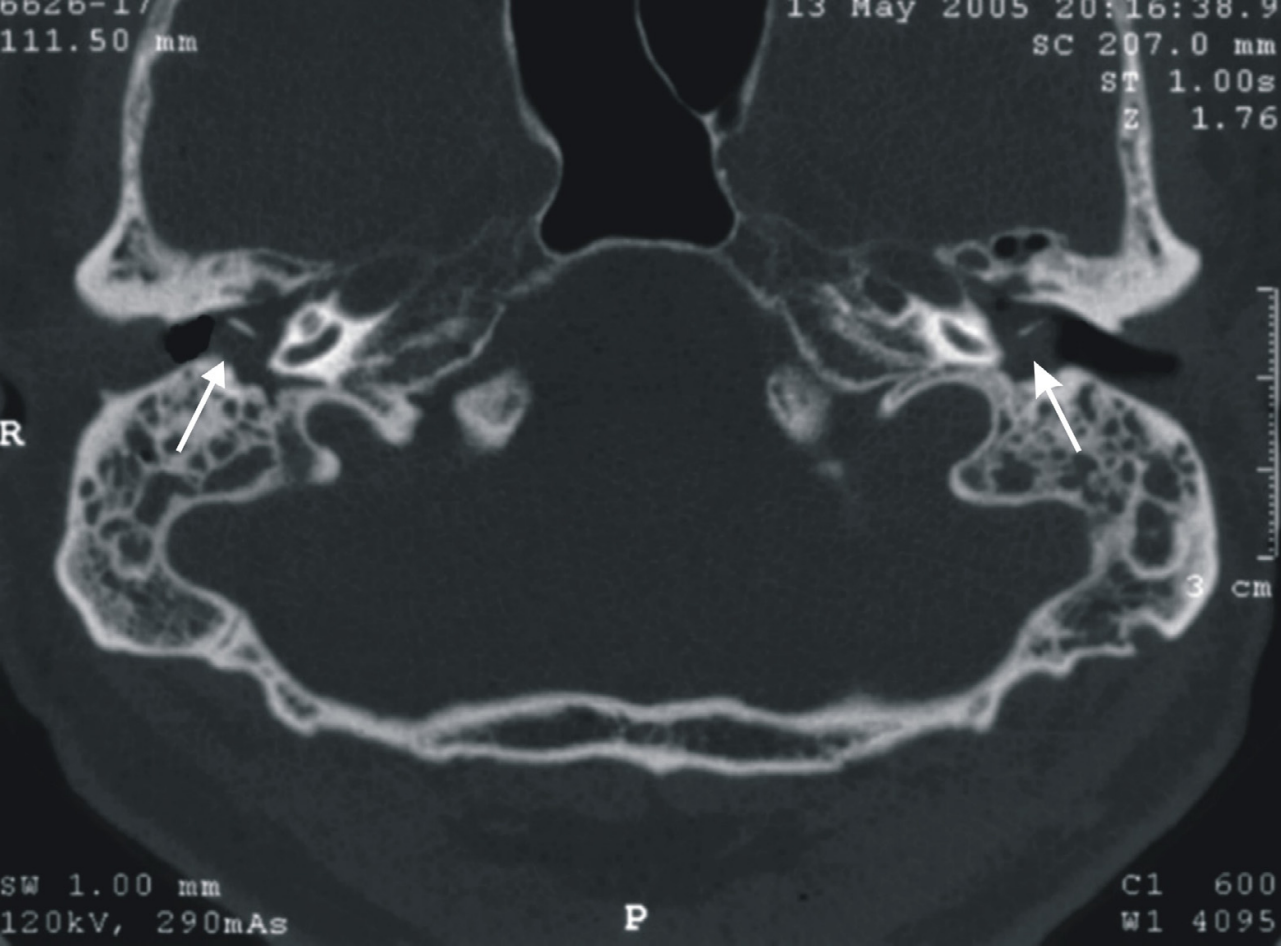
CT scan of the mastoid demonstrating bilateral opacity of the tympanic cavity (white arrows).
